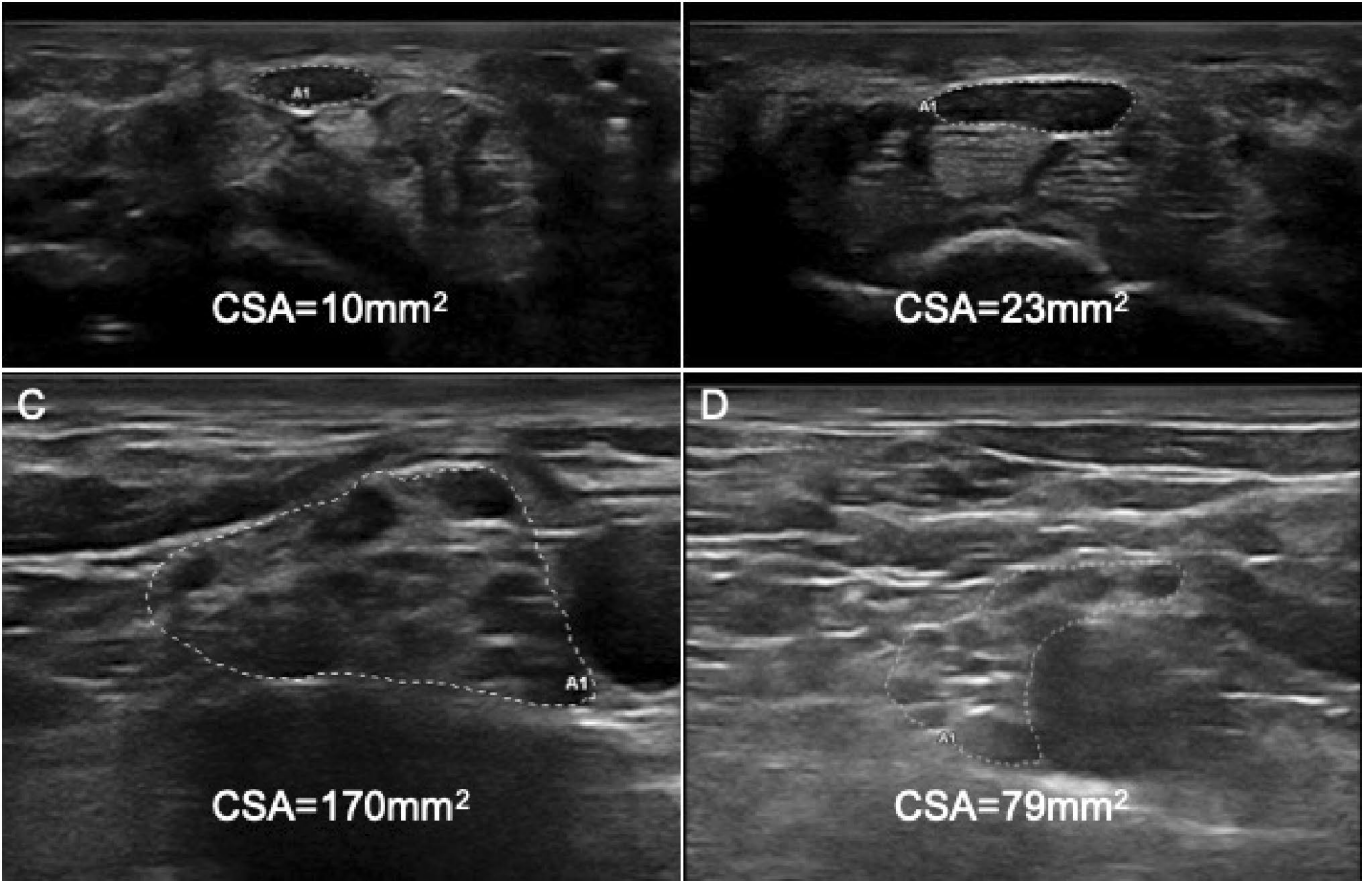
Nerve ultrasound of median nerve at wrist and brachial plexus in supraclavicular space. Panel a shows a severe CTS (grade 4, accordingly to Padua’ scale) in an ATTRv woman, CSA was within normal limit (10mm^2^), while in b an idiopathic CTS of the same severity showed a markedly enlarged CSA (23 mm^2^). In C and D right brachial plexus at supraclavicular space are showed. In c is reported the enlarged plexus of an ATTRv patient (with late-onset Val30Met mutation) with polyneuropathy, in d the normal plexus of the pre-symptomatic son of the same patients. *CSA* Cross sectional area
